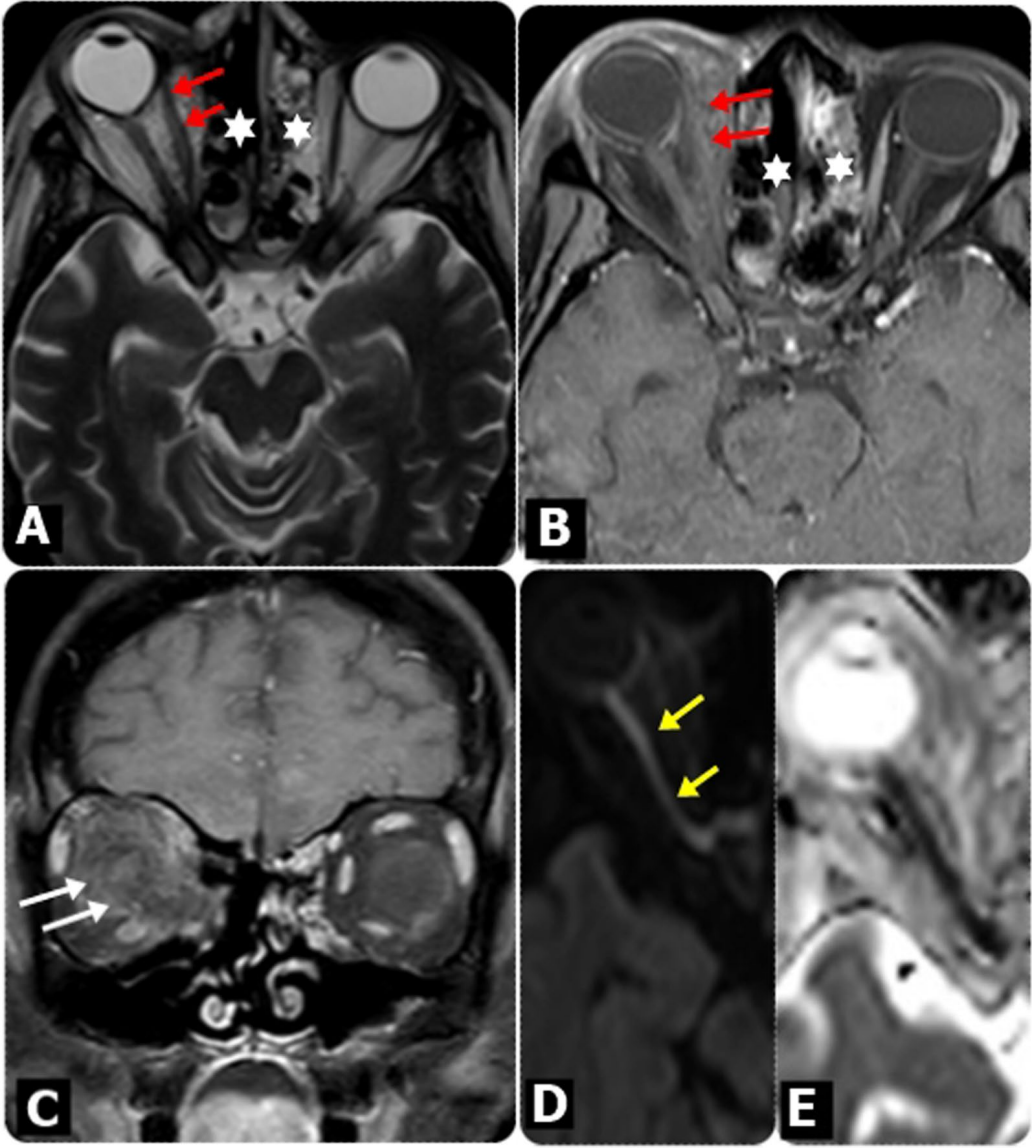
MRI findings in diabetic female patient, 65 years old, presented mainly with Rt. eye proptosis, with decreased in vision, following COVID-19. A Axial T2W as well as B Axial and C Coronal post-contrast fat-saturated T1WI showed bilateral ethmoidal sinusitis (asterisks), with extension of the inflammatory process to the Rt. orbit, resulting in proptosis of Rt. eye globe, with posterior globe tenting, as well as Rt. periorbital and eyelids edema. There is retro-orbital fat stranding (White arrows) and thickened and enhanced Rt. extra-ocular muscles (red arrows). Rt. optic nerve showed restricted diffusion on DW images (Yellow arrows) at b-value 1000 (D) and apparent diffusion coefficient (ADC) map (E) in keeping with Rt. optic nerve infarction
